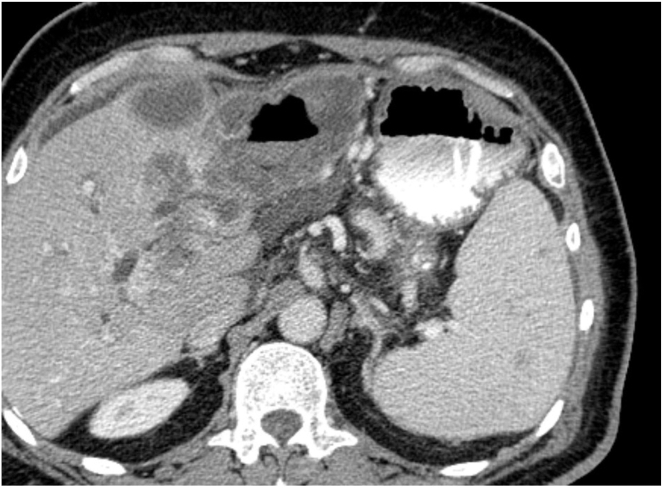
Axial CT sections with oral contrast shows a large cavitary hepatic lesion with positive oral contrast—air level with hyperdense fluid, consistent with fistulization into the gastric antrum.
